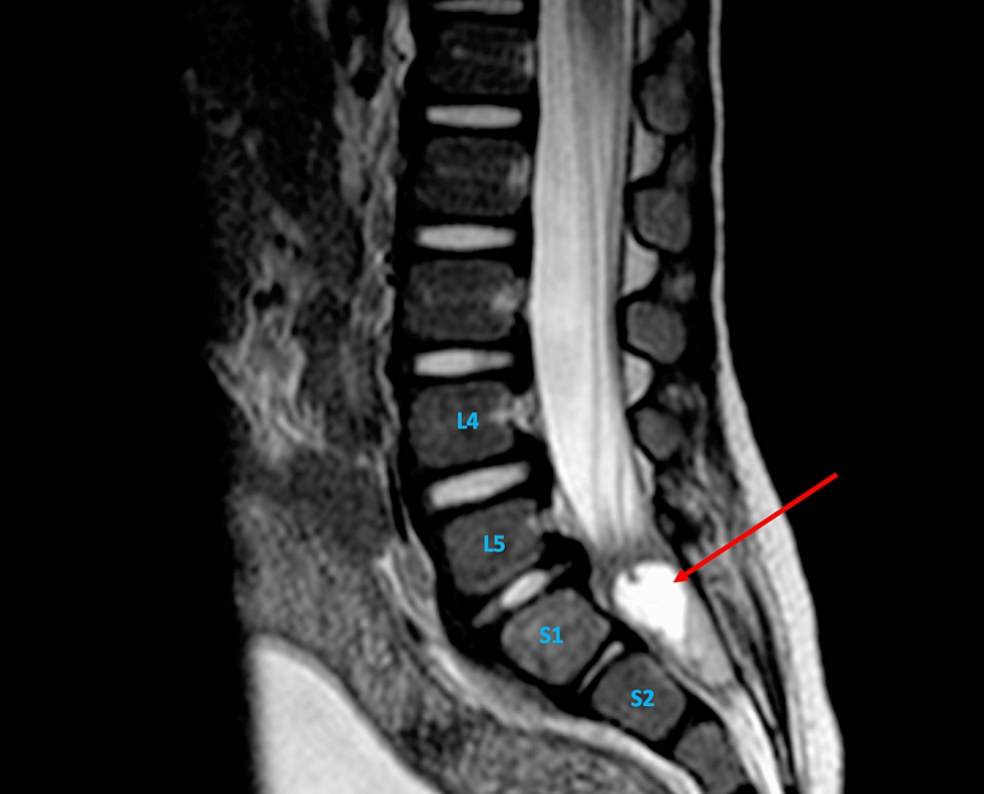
Sagittal MRI of the lumbar spine showing a contrast-enhancing region consistent with fluid collection that measures 2.3×0.9×1.3 cm in size (red arrow) and lies within the epidural space at the level of S1 and S2 vertebrae.
